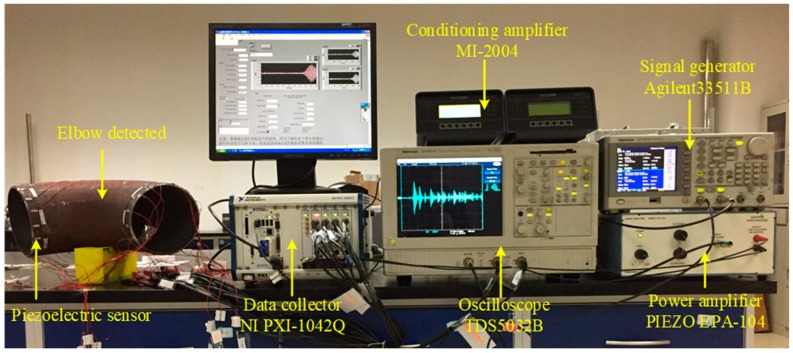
Ultrasonic guided wave detection experimental platform.
